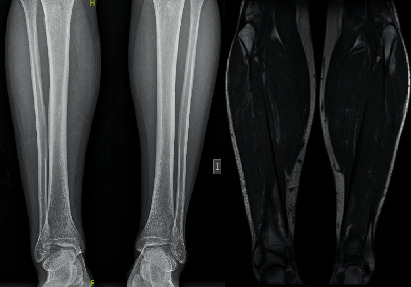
X-ray and MRI study did not show any bony or musculotendinous injury.
